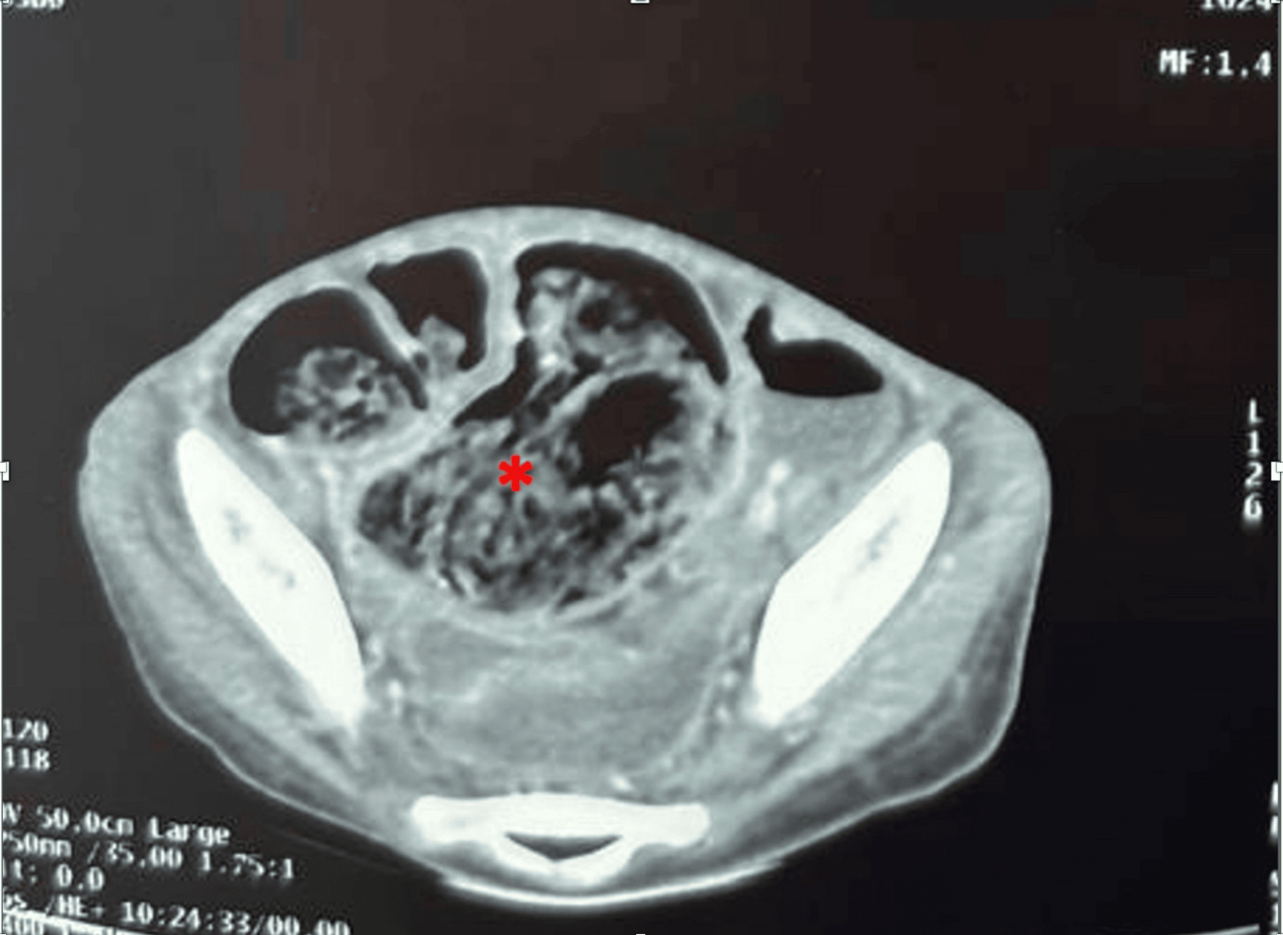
CT abdominal scan showing a pseudo-target-shaped, elongated, dense, and heterogeneous lesion in the distal ileum that does not enhance after contrast agent injection (red star)
